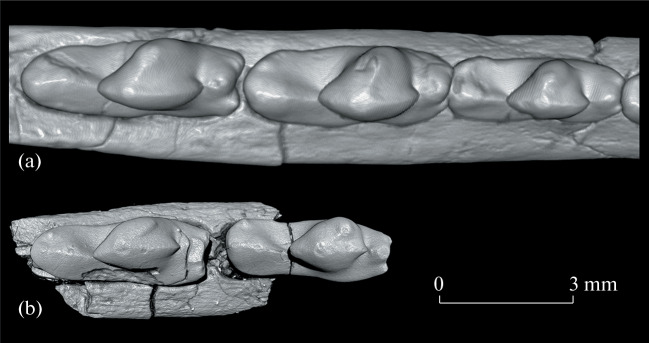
Comparison of the lower molar structure of two species of *Hapalodectes* from the Tsagan-Khushu locality in Mongolia, CT models, occlusal view: (a) *H. dux* Lopatin, 2001, holotype PIN, no. 3104/371, lower jaw, right M_1_–M_3_ region; (b) *H. paradux* Lopatin, sp. nov., holotype PIN, no. 3104/775, right dentary fragment with M_2_–M_3_; Upper Paleocene, Naran Bulak Formation, Zhigden Member.
